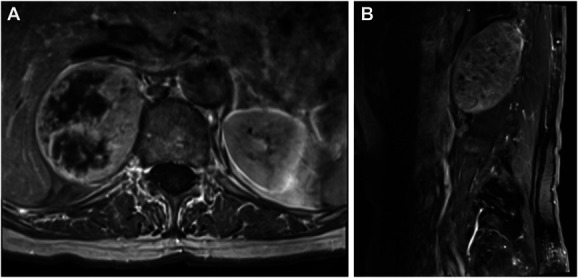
Preoperative T1-MRI with contrast demonstrating a heterogeneously enhancing lesion measuring 8.5 × 5.2 × 6.3 cm in A, axial and B, sagittal views, spanning T11-L2 vertebral bodies.
